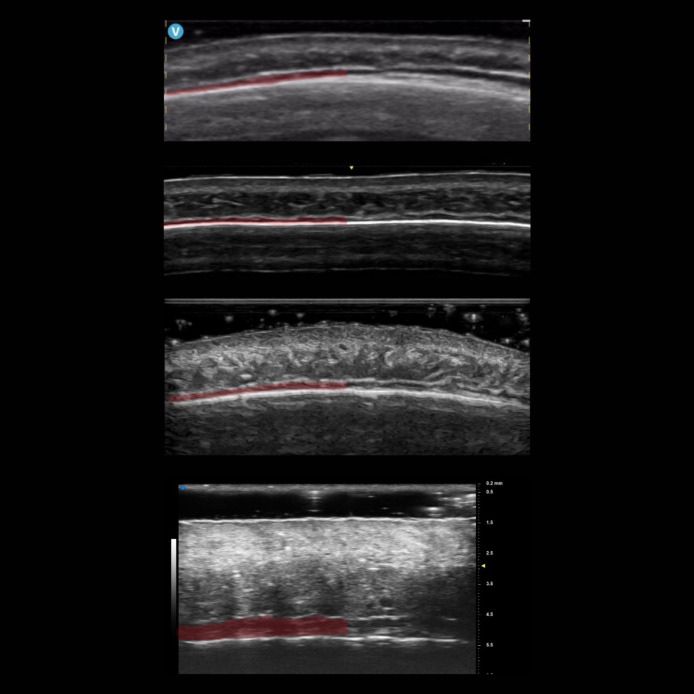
Forehead ultrasound images taken with different devices. Top to bottom: portable linear 12 MHz probe, linear 4-20 MHz probe, linear 6-24 MHz probe, and ultrahigh-frequency 70 MHz probe. The frontalis muscle is marked in red.
